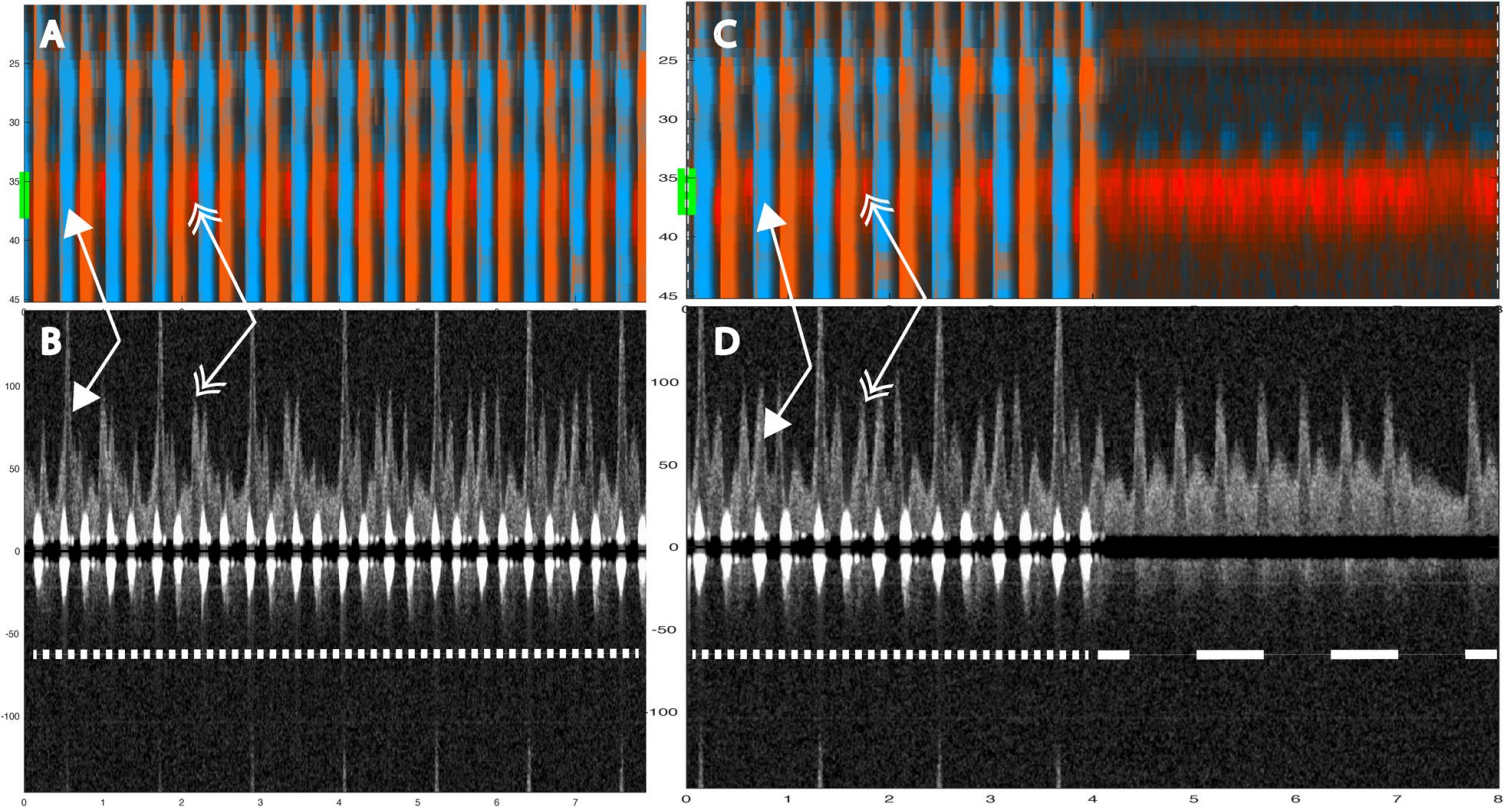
Two 8-s examples showing evidence of spontaneous circulation. In A1 (color M-mode) during manual compressions at 50/ min, one sees spontaneous circulation appearing as red signals at a depth of about 37 mm (yellow line at the A1 and B1 margin). The corresponding Doppler spectrum (A2) from the chosen depth of 37 mm shows spontaneous velocity signals interspersed between the compression-generated spectra. In B1 (color M-mode) during manual compressions, first one sees compression (tissue) artifacts. Spontaneous circulation become evident during the circulatory check. In B2 the spontaneous velocity signals are immediately visible at the circulatory check. Below both panels, a narrow-dashed line indicates ongoing chest compressions, and a wide-dashed line indicates a circulatory check (panel B2). The two double arrows: the single-tip arrows indicate spontaneous circulation (S), and the double-tip arrows indicate chest compression (C). Velocities are in cm/s
